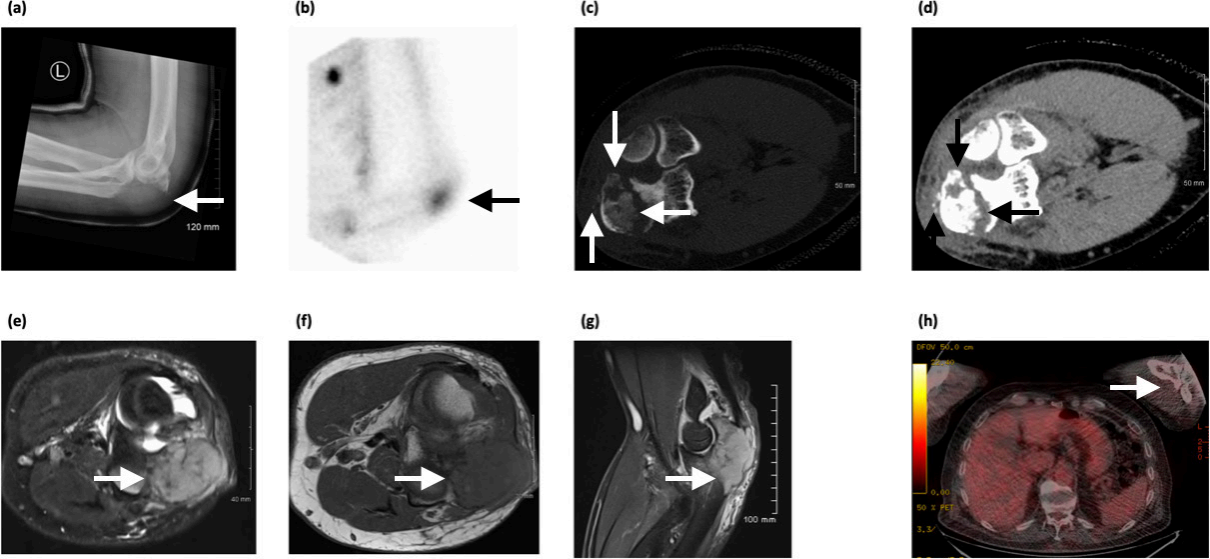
(a) Radiograph of left elbow demonstrating permeative destruction of the left olecranon, (b) bone scintigraphy showing increased radionuclide uptake at corresponding site, (c, d) axial CT image demonstrating typical features of musculoskeletal lymphoma including cortical destruction, extension into the soft tissue and pathological fracture of the olecranon with associated bony fragments, (e) axial *T*_2_ weighted fast spin echo MR image and (f) *T*_1_ weighted MR image in which the lesion is hyperintense (T2) and hypointense (T1) to surrounding muscle, (g) sagittal *T*_1_ weighted turbo inversion recovery magnitude MR image showing hyperintense lesion (arrow), (h) end-of-treatment PET-CT with no evidence of disease in the left elbow. PET, positron emission tomography.
